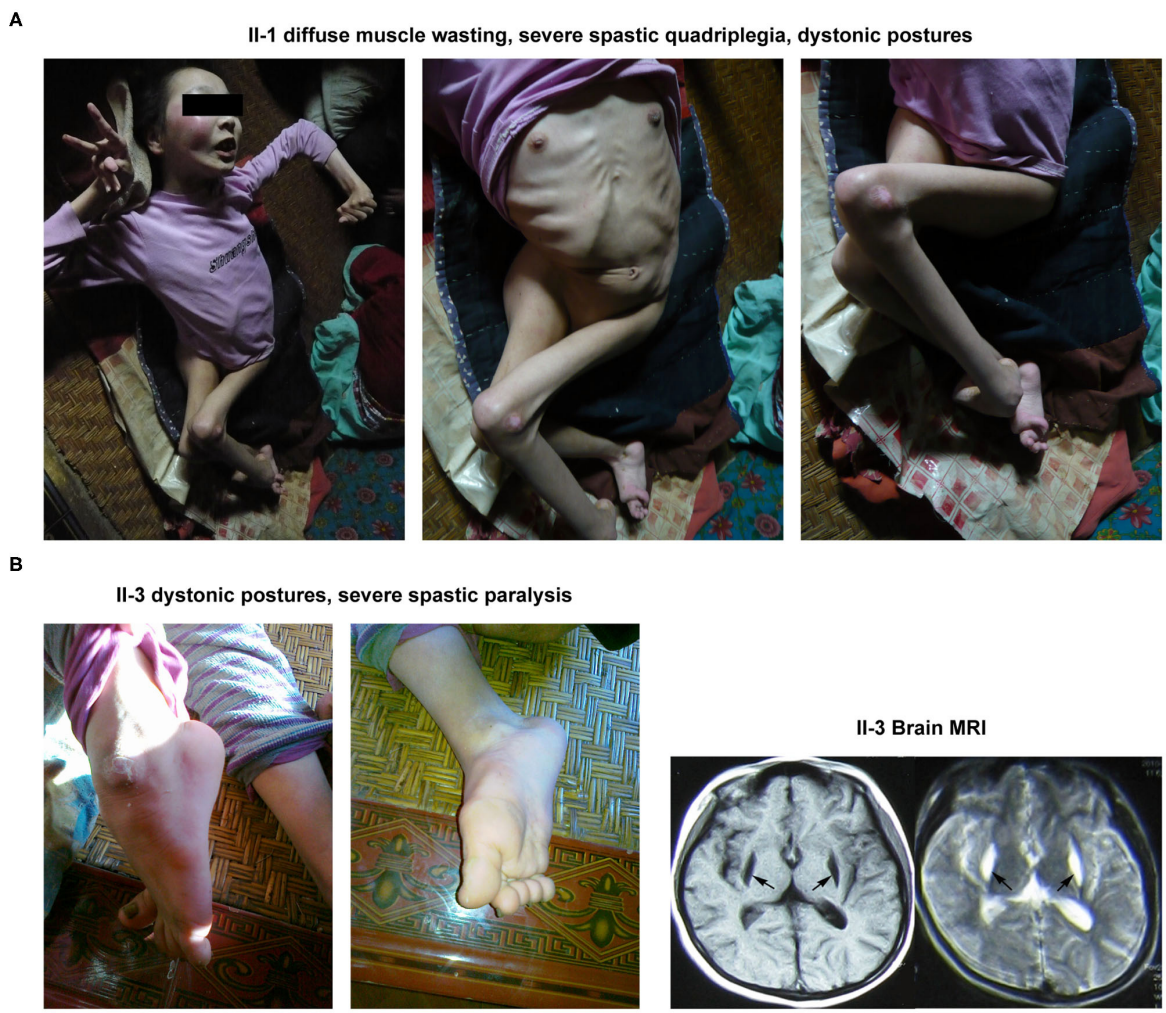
Clinical manifestations of the other 2 patients (II-1 and II-3) in this family. (A) The abnormal appearance of II-1. (B) The dystonic posture in lower limbs and brain MRI result of II-3.
